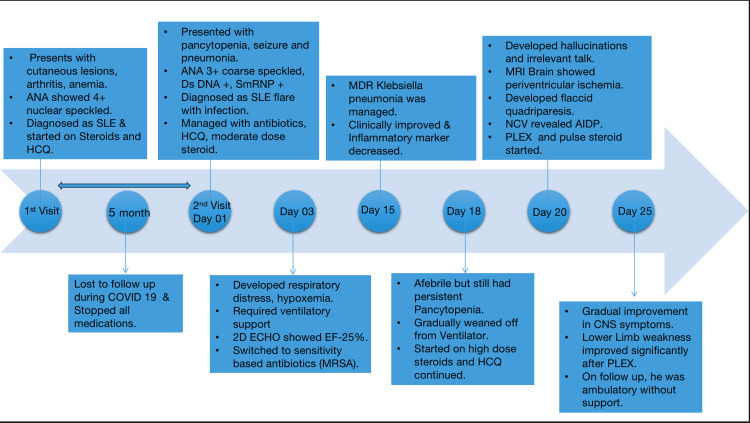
Sequential timeline of various events during the admission period ANA: antinuclear antibody; SLE: systemic lupus erythematosus; HCQ: hydroxychloroquine; dsDNA: double-stranded deoxyribonucleic acid; MDR: multi-drug resistant; MRSA: methicillin-resistant Staphylococcus aureus; MRI: magnetic resonance imaging; NCV: nerve conduction velocity; AIDP: acute inflammatory demyelinating polyneuropathy; PLEX: plasma exchange
